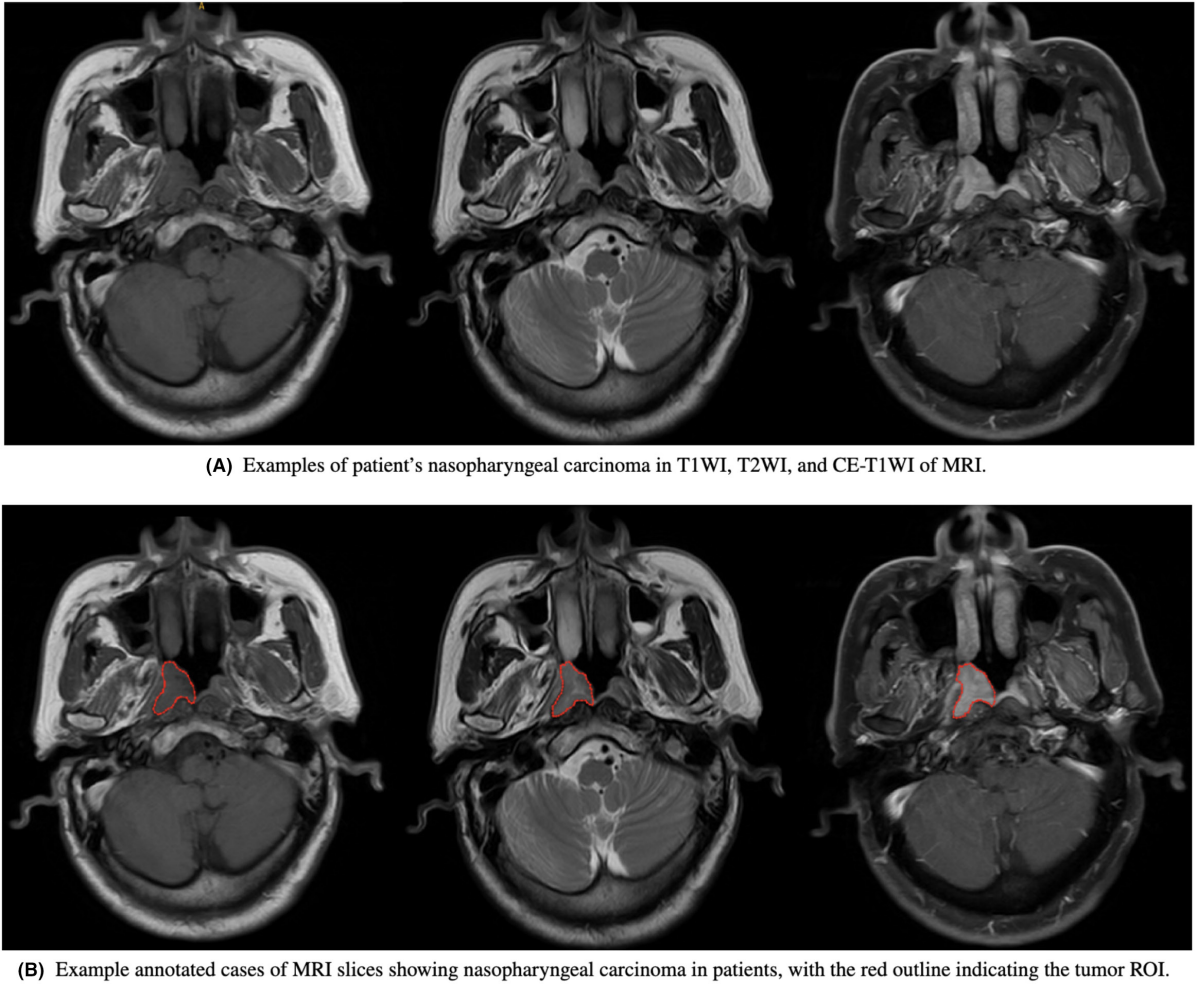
An example of MRI slices from a patient with nasopharyngeal carcinoma, with T1WI, T2WI, and CE‐T1WI images arranged from left to right.
